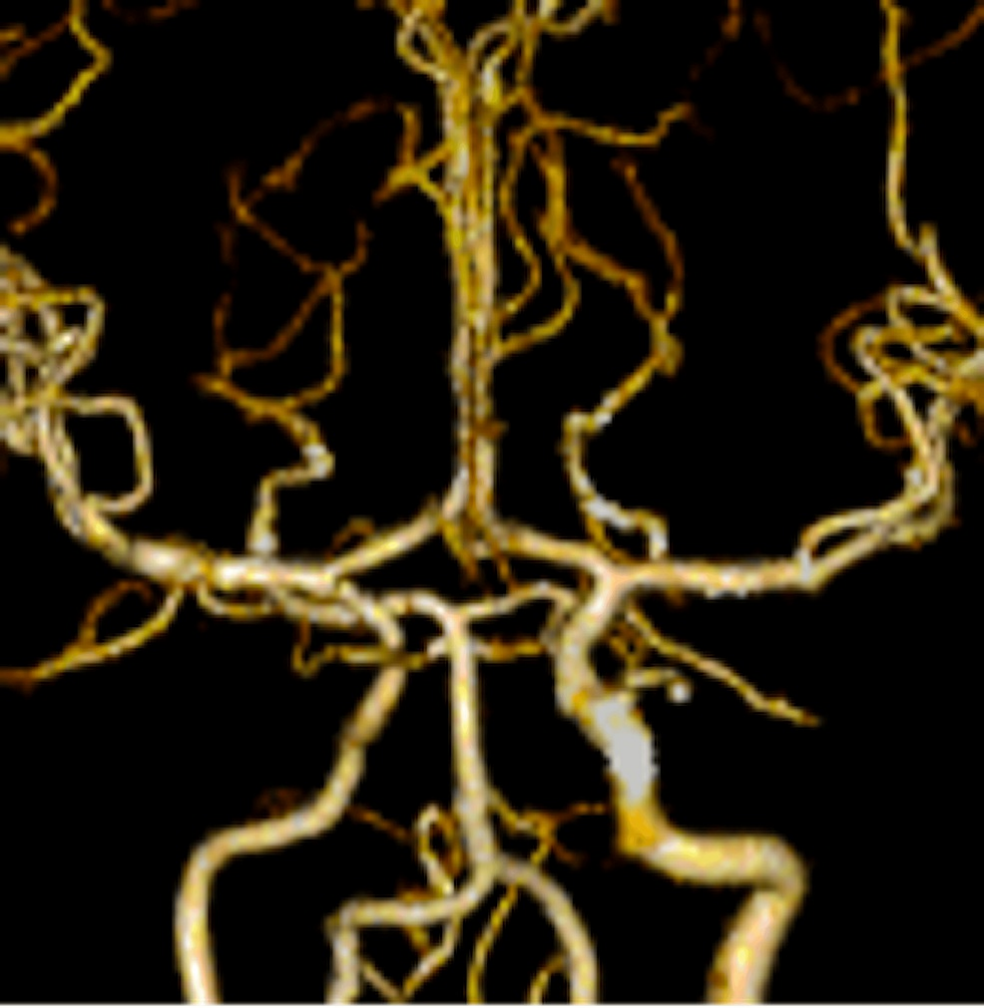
A CT angiography on the 29th day showed an almost normal shape of MCA. CT, computed tomography; MCA, middle cerebral artery
